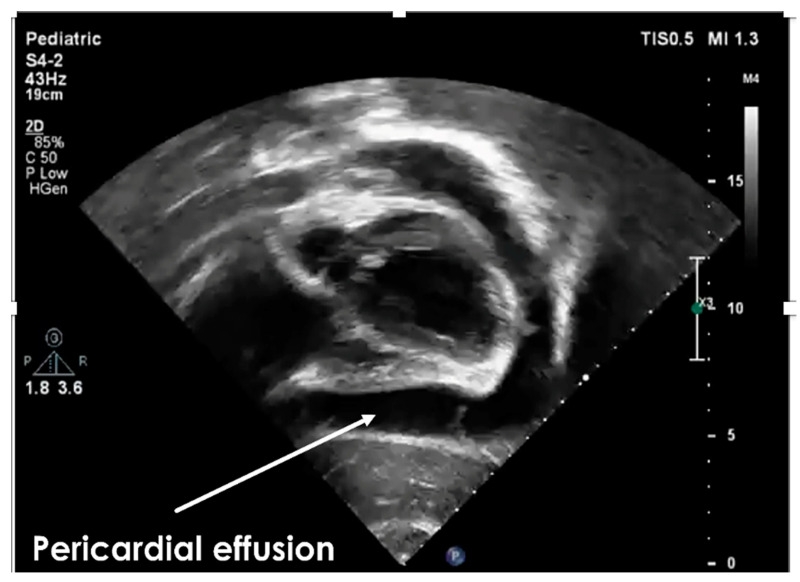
The echocardiographic subcostal view during the first presentation of our subject for acute pericarditis showed moderate pericardial effusion. Legend: white arrow showing pericardial effusion.
